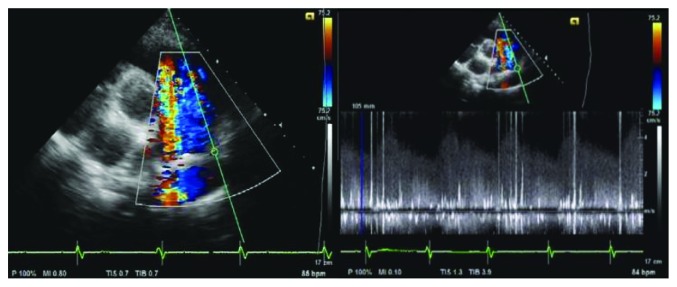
In the short axis view at the level of the aortic valve, there is a retrograde mosaic jet entering the distal pulmonary artery from the posterolateral direction, indicative of patent ductus arteriosus, with the following measurements: PSAX 9 mm, 10 mm (with color); suprasternal 8.1 mm, 1 mm (with color). Continuous flow across the defect is demonstrated on spectral Doppler. Computed Qp : Qs is 1.18.
